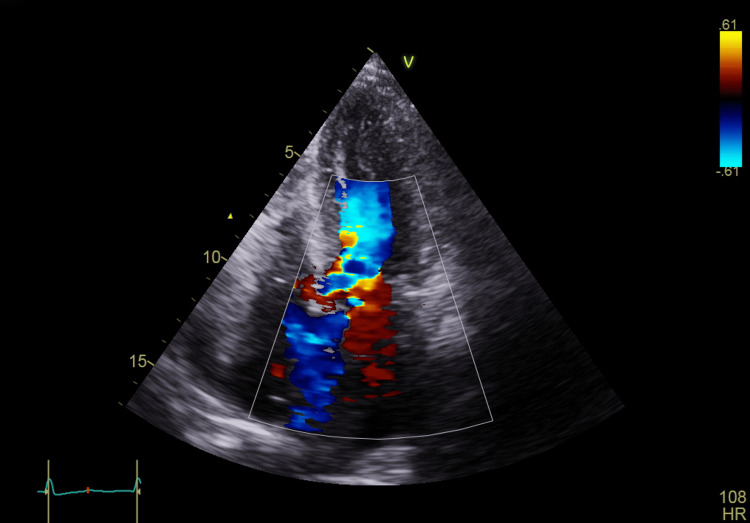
Apical four-chamber view showing LVOT obstruction with high-velocity turbulent flow LVOT: left ventricular outflow tract
